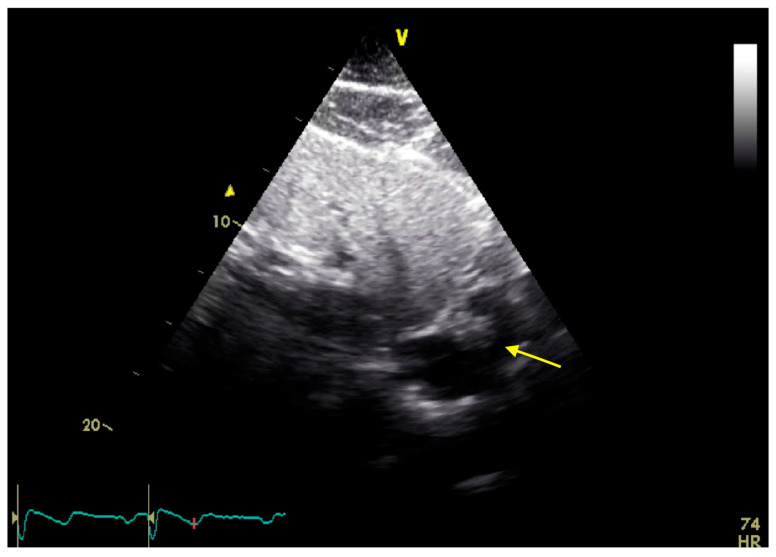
Transthoracic echocardiography in a subcostal view showing the CAT (yellow arrow).
